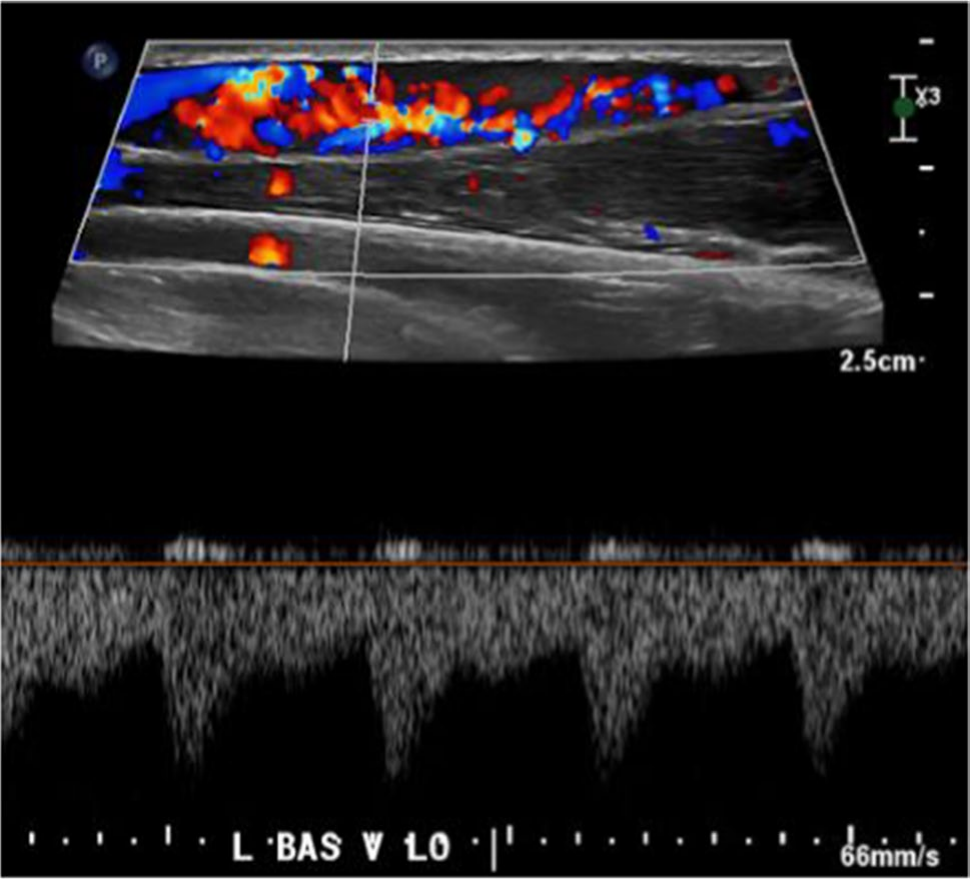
At the time of biopsy, there is increased vascularity with low resistance arterial waveform within the mass on longitudinal spectral Doppler ultrasound images at time of biopsy
